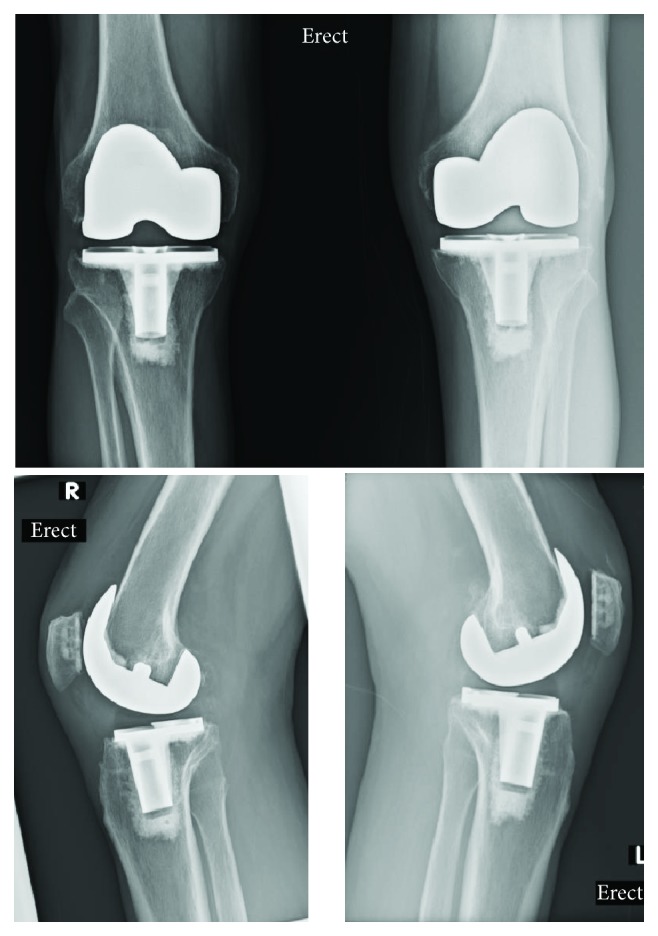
Postoperative X-rays of the knees.
